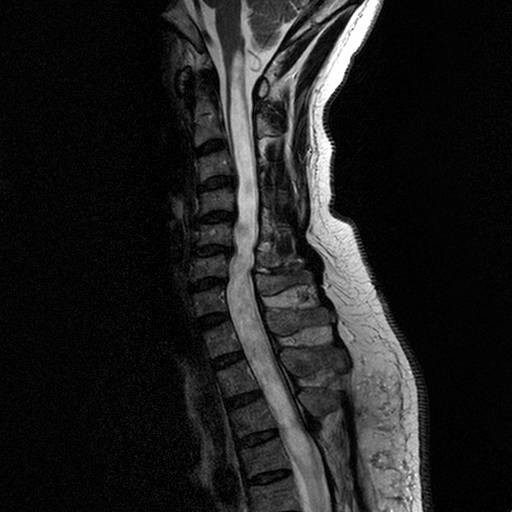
Sagittal magnetic resonance image of the cervical spine showing the syrinx. Although tubular hypointensity is observed on T2-weighted images, there is no connection with the fourth ventricle.
